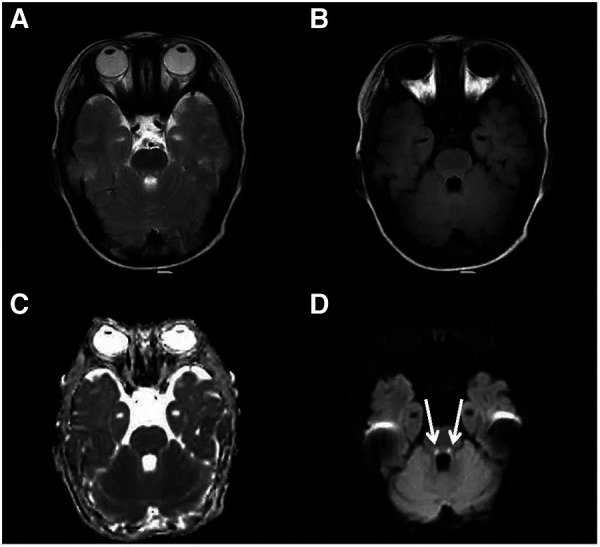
Cranial MRI at the level of the dorsal pons. An axial T2-weighted (A), an axial FLAIR (B), a dADC (C) and a DWI (D) scans are shown and abnormally high signals in DWI in the dorsal pons can be seen (white arrow).
